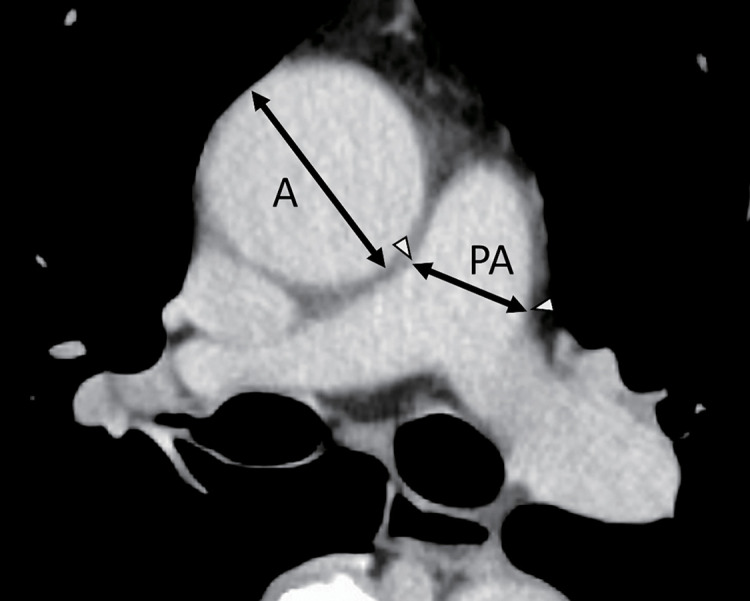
PA and aorta (A) diameter measurements; chest CT axial image was used, and the measuring point of the main PA is the PA bifurcation; ascending aorta diameter was measured at the same CT slice as the PA measurement; red arrowheads show the bifurcation of the bilateral pulmonary arteries, and black arrows show the PA and A diameter to calculate the PA/A ratio. A: aorta; CT: computed tomography; PA: pulmonary artery; PA/A ratio: pulmonary artery to aorta diameter ratio
